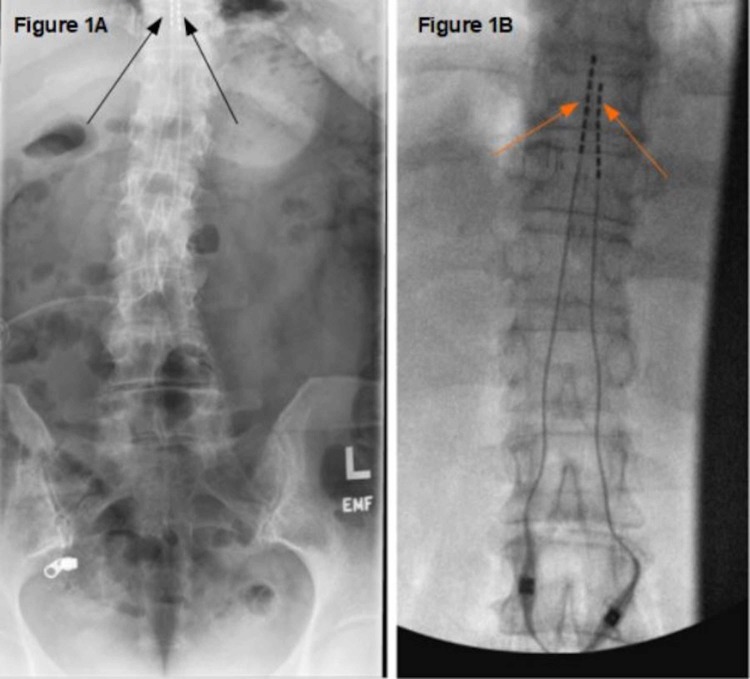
Preoperative and postoperative pictures depicting old and new spinal cord stimulator apparatus (A) Preoperative lumbar radiograph with two percutaneous leads at T9/10 (Black arrows). (B) Postoperative placement of two percutaneous leads at T8/T9 (Orange arrows).
